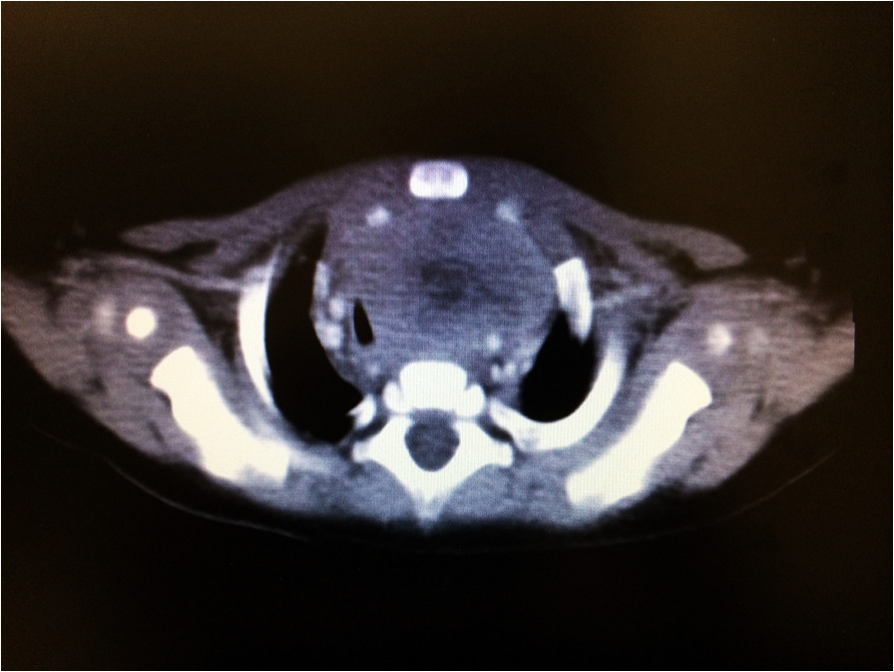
Computed tomographic view of the anterior mediastinal mass compressing and displacing the trachea and great vessels
